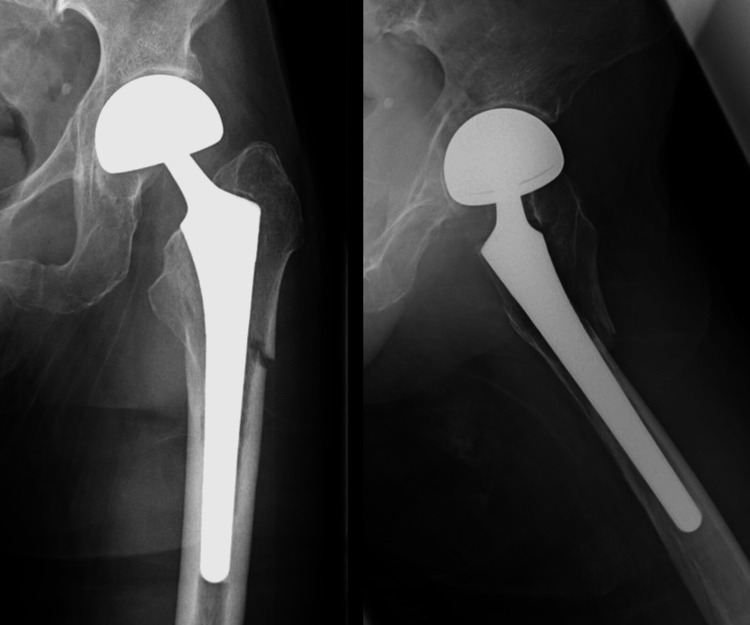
Preoperative radiographs of case 3 Preoperative radiographs of case 3 showed PFF of Vancouver classification Type B1. PFF: periprosthetic femoral fracture.
